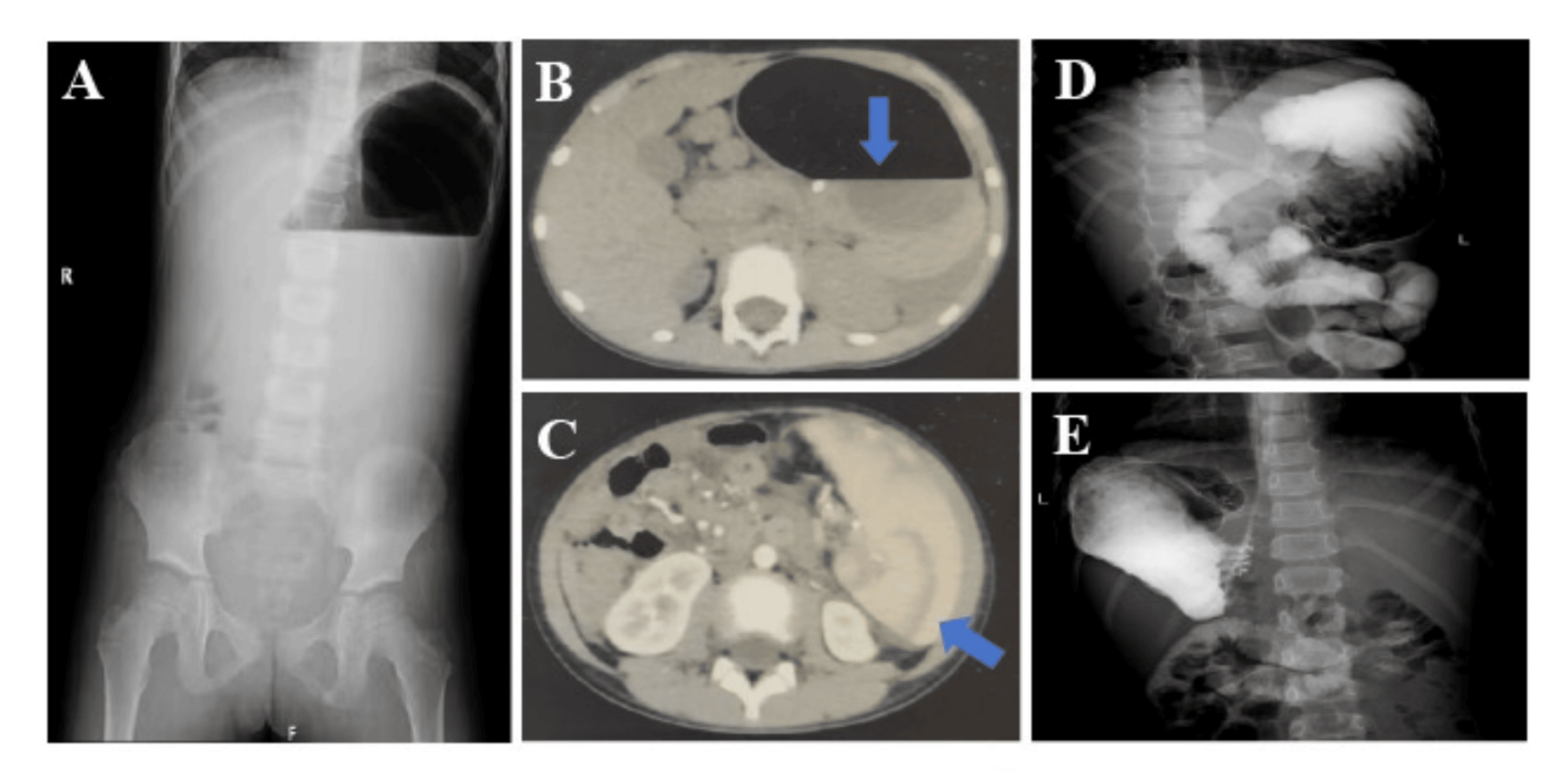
Abdominal image finding (A) Upright abdominal plain film demonstrated gastric outlet obstruction with dilatation and stasis. (B) Abdominal CT showed a distended stomach with retained contents (blue arrow). (C) The CT highlighted significant splenomegaly (blue arrow). (D-F) Upper gastrointestinal series revealed a complete 180° organoaxial gastric volvulus (the greater curvature situated above the lesser curvature). CT: computed tomography
